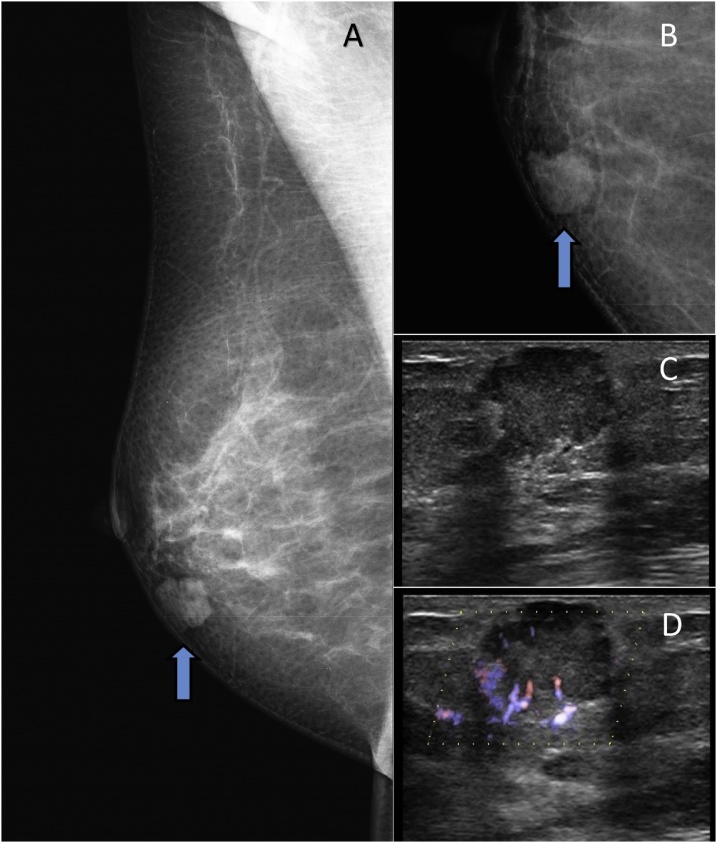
Breast imaging. Mammography suggested a lobulated, radiopaque lesion with mostly sharp contours and hallo sign (A,B). Breast ultrasonography revealed an round-shaped, low-echoic tumor of unclear etiology (C) with a pathological pattern of blood flow, as seen on Power-Doppler imaging (D).
